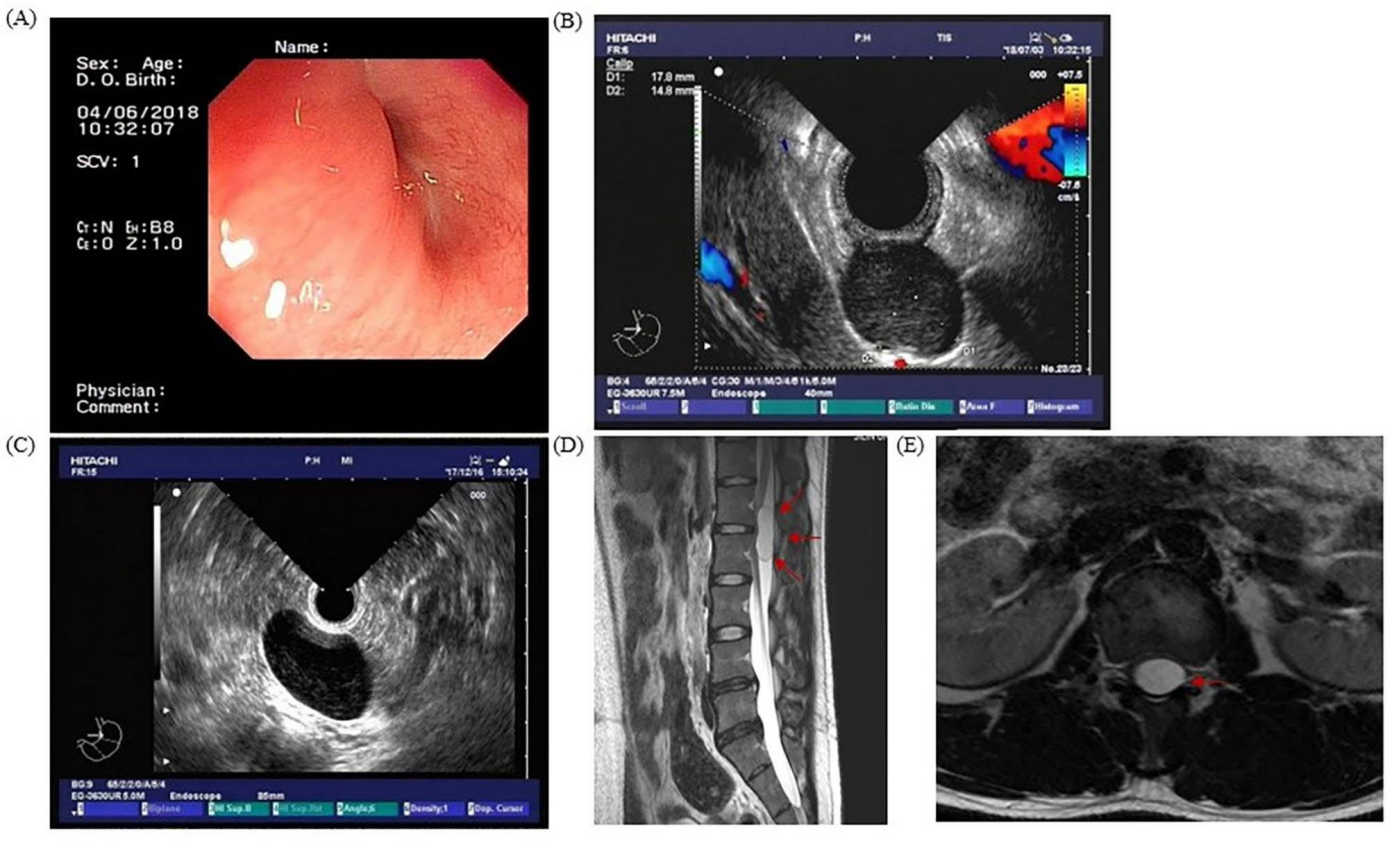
Representative images of patients diagnosed with BCs. (A, B), Case No. 1 with the post-operative diagnosis of a diaphragmatic bronchogenic cyst. Pre-operative endoscopic examination revealed a slight mucosal bulge in the lower esophagus near the dentate line (A). A well-defined oval hypoechoic mass with homogeneous internal echoes outside the wall of the bulging esophagus (13.9*19.6 mm in cross-section) was detected via the subsequent ultrasound microprobe examination and was suspected to be attached to the intrinsic muscular layer of the esophagus (B); (C), Case No. 2 with a post-operative diagnosis of a retroperitoneal bronchogenic cyst. Pre-operative ultrasound endoscopy found a smooth cystic mass outside the gastric wall without an echogenic signal (29.9 * 38.8 mm in cross-section); (D, E) Case No. 3 with a post-operative diagnosis of an intraspinal bronchogenic cyst. Pre-operative magnetic resonance imaging of the lumbar spine showed a cystic occupying lesion in the spinal canal near the T1 and T2 vertebrae (red arrows)
